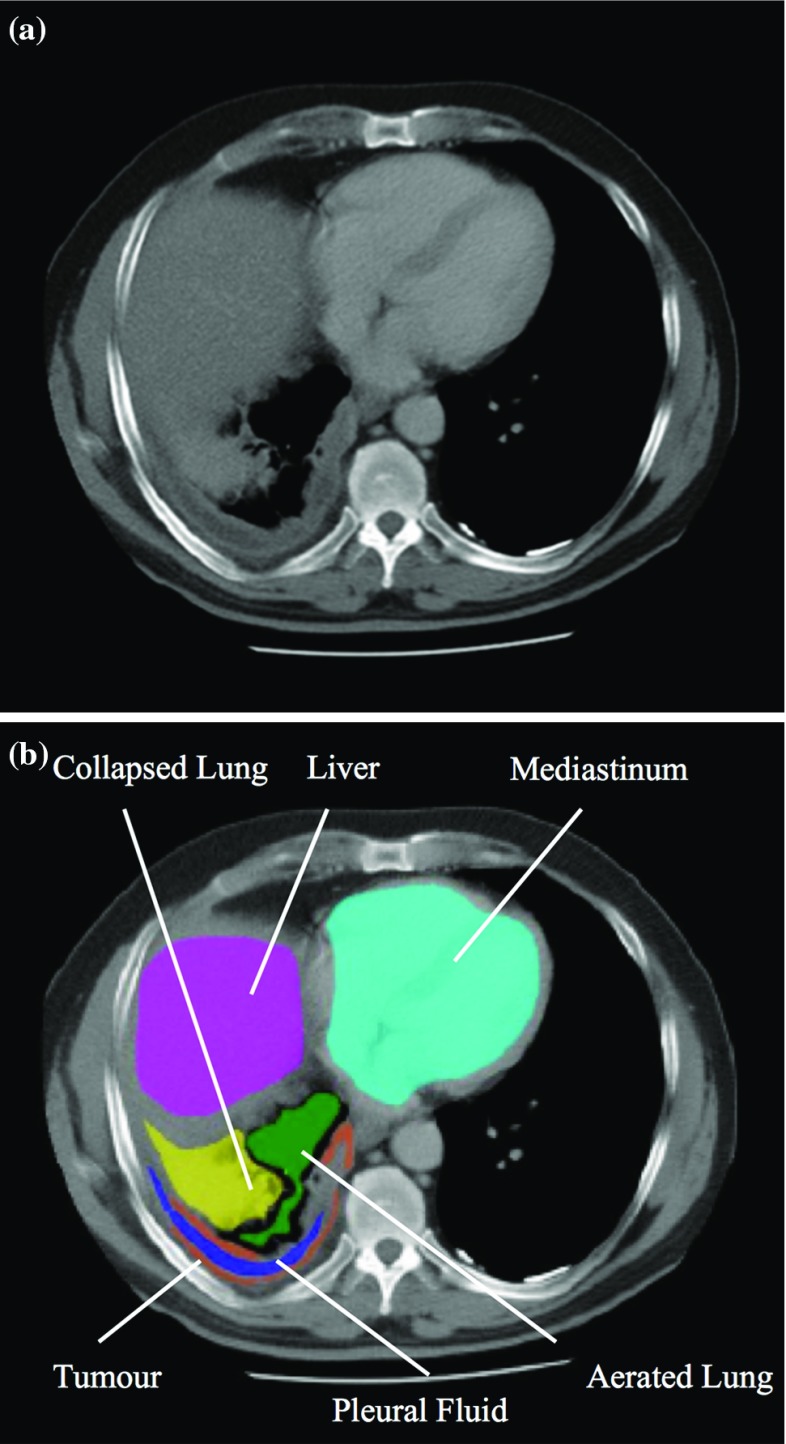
Sample CT image slice with key regional tissues *highlighted*. Tumour is shown in *orange*. a Original, b segmented CT
